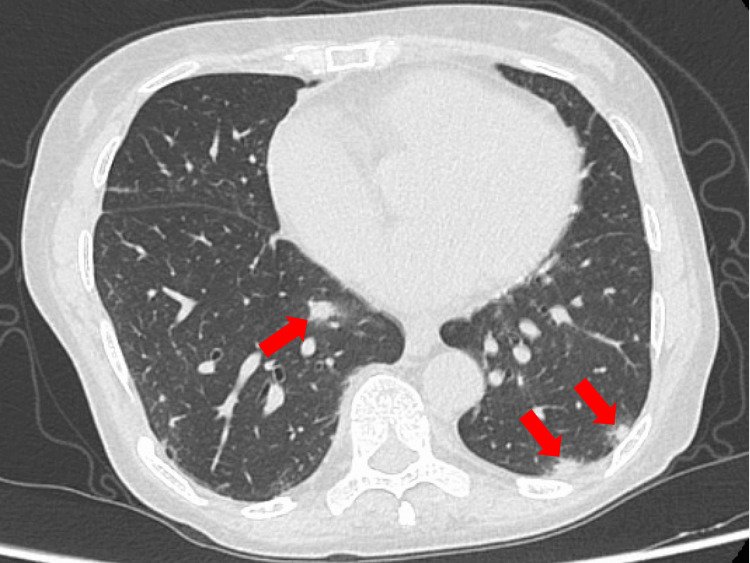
Chest computed tomography imaging on the day of admission Chest computed tomography revealed multiple micronodules in both lungs.
